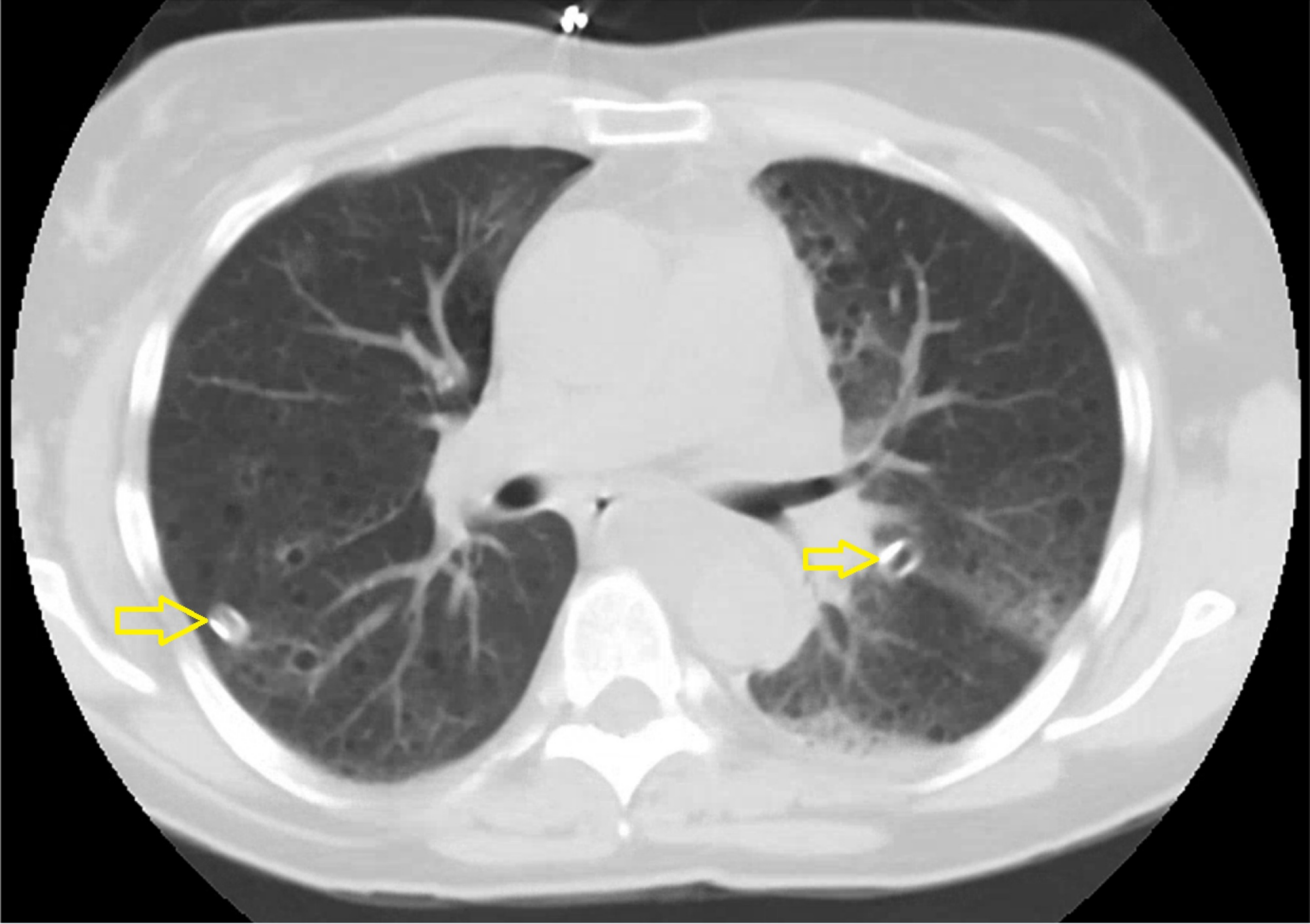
CT chest without contrast showing bilateral chest tubes (yellow arrowhead) with a near-complete resolution of bilateral pneumothoraces.
